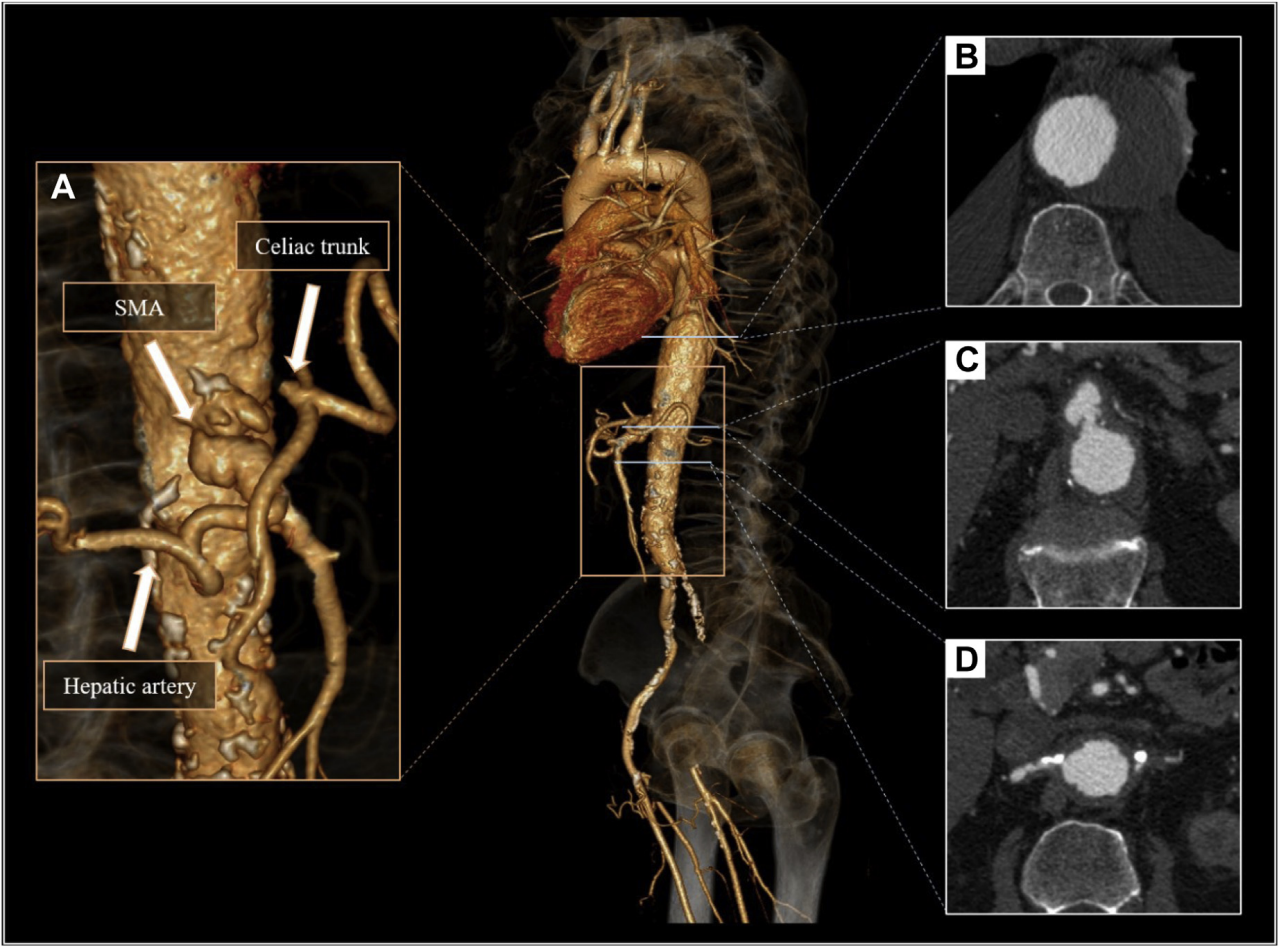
Preprocedural computed tomography (CT) angiography. A, Volume rendered image of the aorta displays the pseudoaneurysm of the superior mesenteric artery (*SMA*), aberrant right hepatic artery, and the occlusion of the celiac trunk. B, Thoracoabdominal aneurysm with a maximal diameter of 70 mm. C, Ostial pseudoaneurysm of the superior mesenteric artery. D, Occlusion at the origin of the left renal artery.
